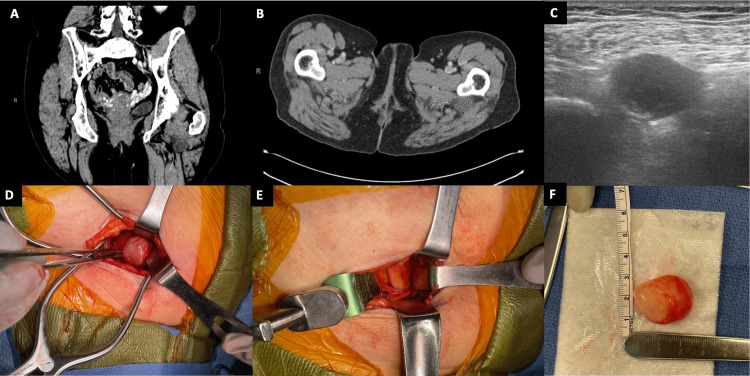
Case 3 preoperative and intraoperative imaging CT-contrasted scan of pelvis with axial (A) and coronal (B) imaging demonstrating an approximately 3x2.4x2.1cm lesion. (C) Intra-op ultrasound of the lesion. Series of intra-op imaging demonstrates the lesion in-situ (D), as well as the sciatic nerve after resection (E), and the lesion post-excision (F).
